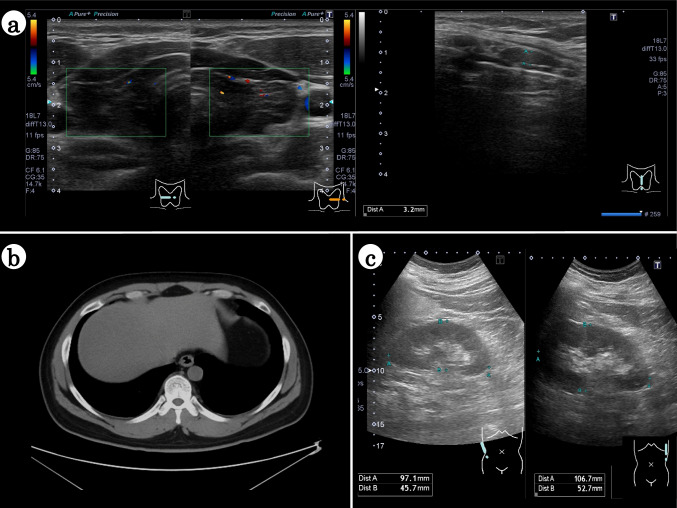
US and CT findings. a US images of the thyroid show diffuse swelling and a hypoechoic thyroid. No blood flow signal enhancement was observed. b Hematological examination shows liver dysfunction, but liver CT shows no abnormalities in liver morphology. c US images of bilateral kidneys show no indications of kidney dysfunction. The patient’s kidneys show a normal morphology and no atrophy. US: ultrasound, CT: computed tomography
